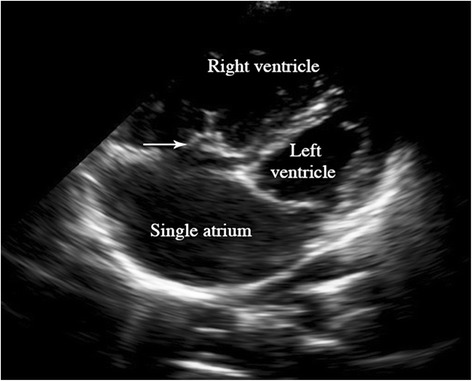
Echocardiographic image. An echocardiogram revealed single atrium and tricuspid valve prolapse (*arrow*)
